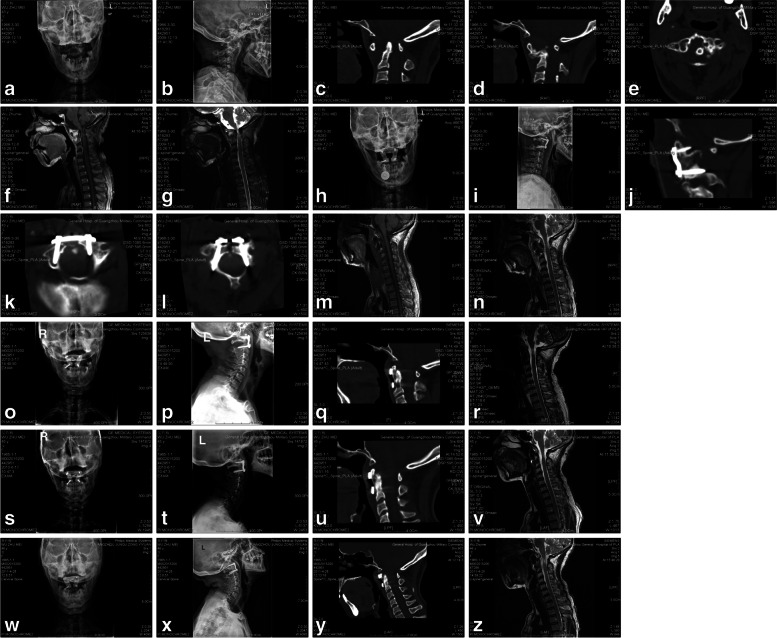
Shows the examination results of a 43-year-old female with basilar invagination, atlantoaxial dislocation and neurofibromatosis. She was treated with transoral atlantoaxial reduction, antogenous iliac bone-grafting and internal fixation with the help of transoral atlantoaxial reduction plate. As shown in a and b, the X-rays reflect obvious anterior atlantoaxial dislocation. The sagittal CT (see c) further shows that odontoid tip is 2.5 mm higher than the Chamberlain line, which can be considered as basilar invagination. According to d and e, the sagittal and axial CTs (near the left lateral mass) show callus hyperplasia in the atlanto-dental interval (ADI) space, leading to the irreducible atlantoaxial dislocation. MRI results (see f and g) show significant compression of cervical medulla at atlantoaxial level and partial degeneration of spinal cord. The postoperative X-rays (see h and i) immediately show that the atlantoaxial vertebra has been completely reducible, and the internal fixation is in good position. According to j, k and l, the postoperative CTs immediately show good position of the atlantoaxial screw path, no invasion of vertebral artery and spinal canal, and the bony callus in the ADI space is removed. The postoperative MRI results (see m and n) demonstrate that there is no significant compression of cervical spinal cord at atlantoaxial level, and no significant changes in partial degeneration of spinal cord. X-rays (see o and p) conducted 3 months after the operation show that the internal screw fixed in the atlantoaxial segment is in place without obvious loosening or displacement. The CT (see q) shows callus formation in the ADI, and initial osseous fusion of the atlantoaxial segment. The MRI (see r) shows that there is no significant compression of cervical spinal cord and no significant change in partial degeneration of spinal cord. X-rays which were conducted 6 months (see s and t) and 17 months (see w and x) after the operation demonstrate that the atlantoaxial internal fixation is firm without any obvious dislocation. In addition, the physiological curve of cervical spine is good, and there is no instability of adjacent segments. The CTs conducted 6 months (see u) and 17 months after operation (see y) show further callus formation in the ADI space, and firm osseous fusion. The MRIs conducted 6 months (see v) and 17 months (see z) after operation show no significant compression of cervical spinal cord. In addition, partial degeneration of spinal cord shows some improvement
